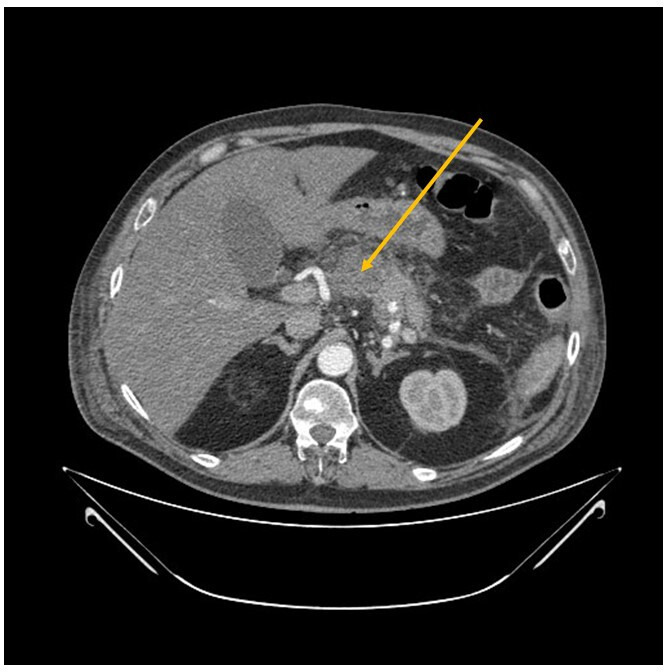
Forty-eight hour CT scan showing acute pancreatitis and peripancreatic fluid with no hypodense areas of pancreatic necrosis (yellow arrow).
